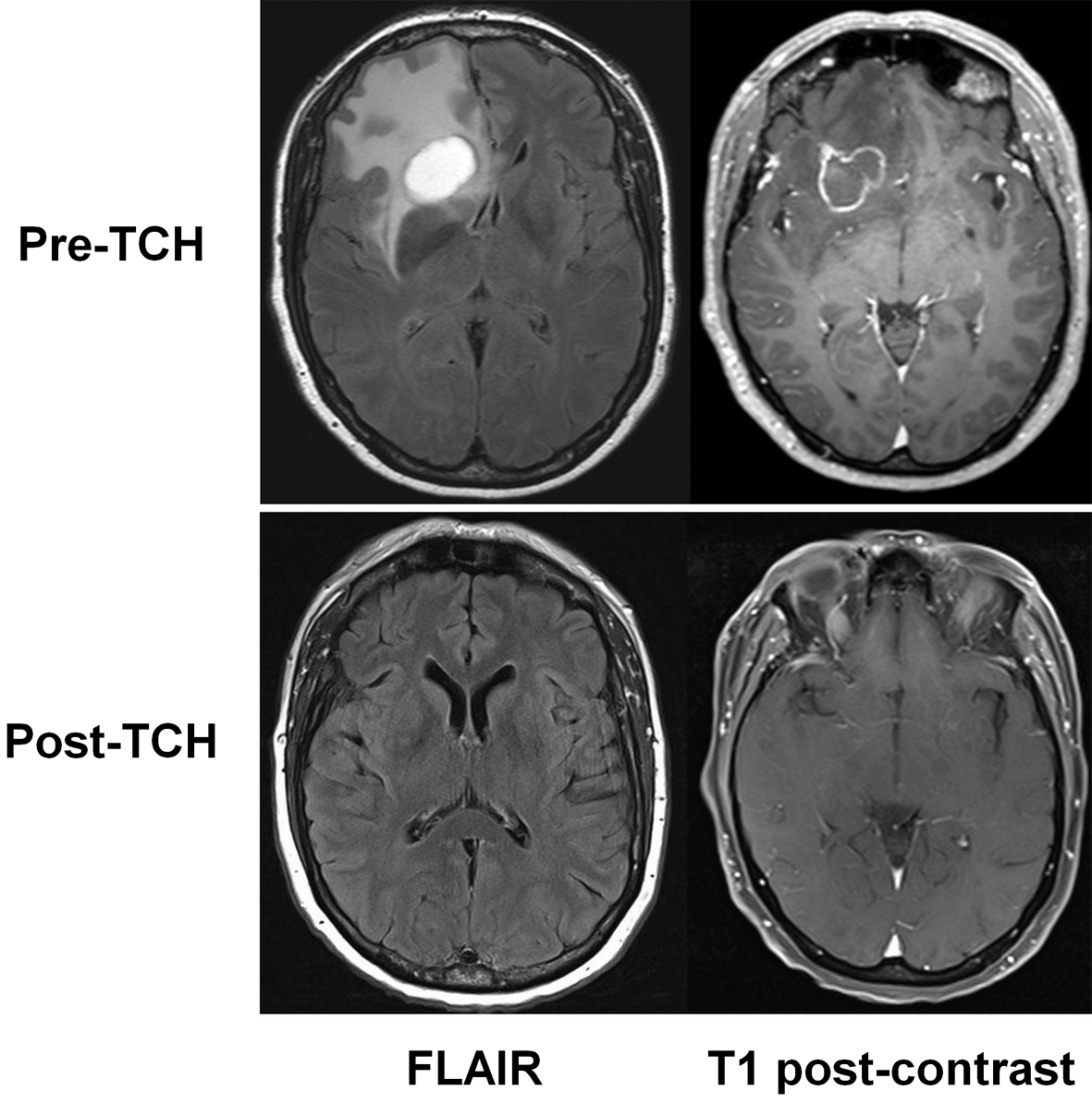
MRI appearances of brain metastases before (pre-TCH, above) and after drug treatment (post-TCH, below). Fluid attenuated inversion recovery (FLAIR) images are shown at left, and T1-weighted images at right.
